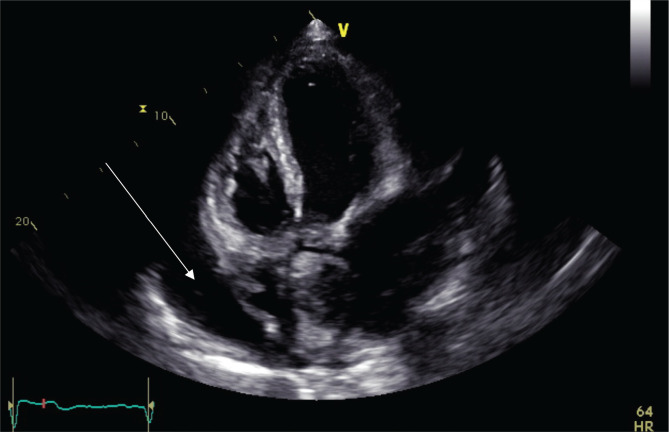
Echocardiography, apical four chambers view.
